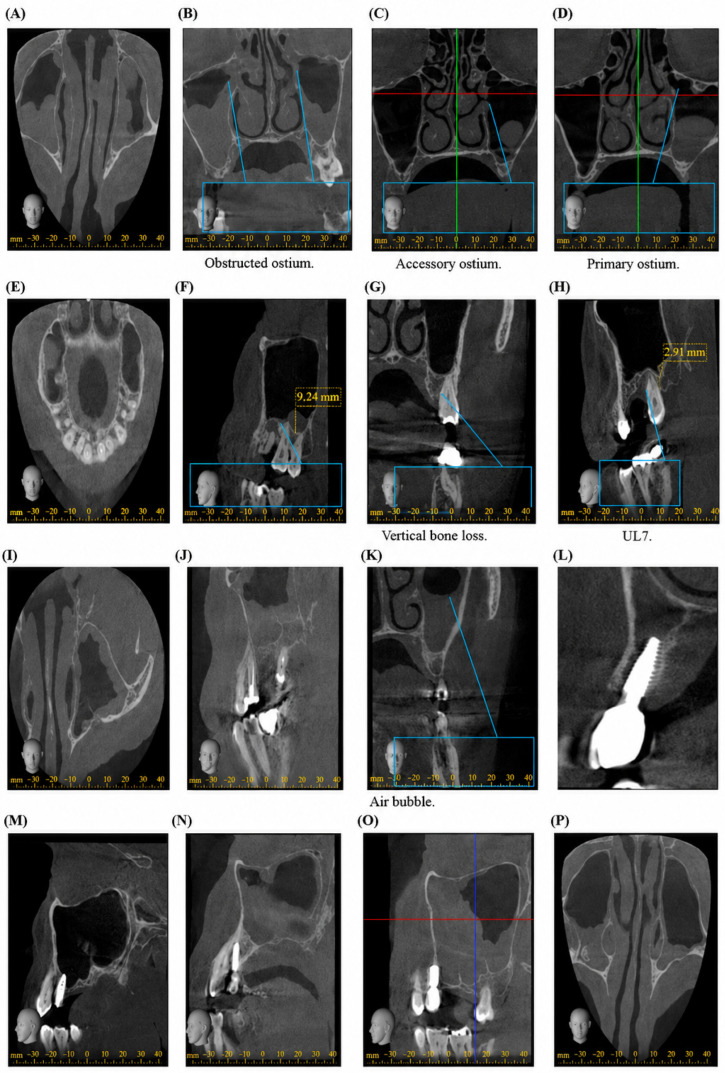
CBCT sections of sinuses that would warrant onward referral based on the framework by Janner et al. (2020) [18]. (A). Left maxillary sinus with an air–fluid level occupying >50% sinus; the ostium cannot be seen. (B). Polypoid lesion occupying the left maxillary sinus exceeding 50% sinus volume. (C). Bilateral sinuses with polypoid thickening occupying >50% sinus volume with bilateral ostium obstruction. (D–F) One right and two left maxillary sinuses with circumferential thickening and ostium cannot be visualised. (G,H). Coronal and sagittal CBCT views showing vertical bone loss at UL7 with flat sinus floor thickening of 2–5 mm. (I,J). Axial and sagittal CBCT views showing periodontal disease and root-filled teeth with circumferential polypoid thickening >10 mm. (K,L). Coronal and sagittal CBCT views showing an air–fluid level associated with a UL4 implant breaching the sinus floor. (M). Sagittal CBCT view showing a UL4 implant breaching the sinus floor without mucosal thickening. (N,O). Sagittal CBCT views showing implants UR5 and UL4 with bilateral polypoid circumferential thickening >10 mm and bilateral ostium obstruction. (P). Axial CBCT view showing an antrolith in the superior anterolateral aspect of the right maxillary sinus.
